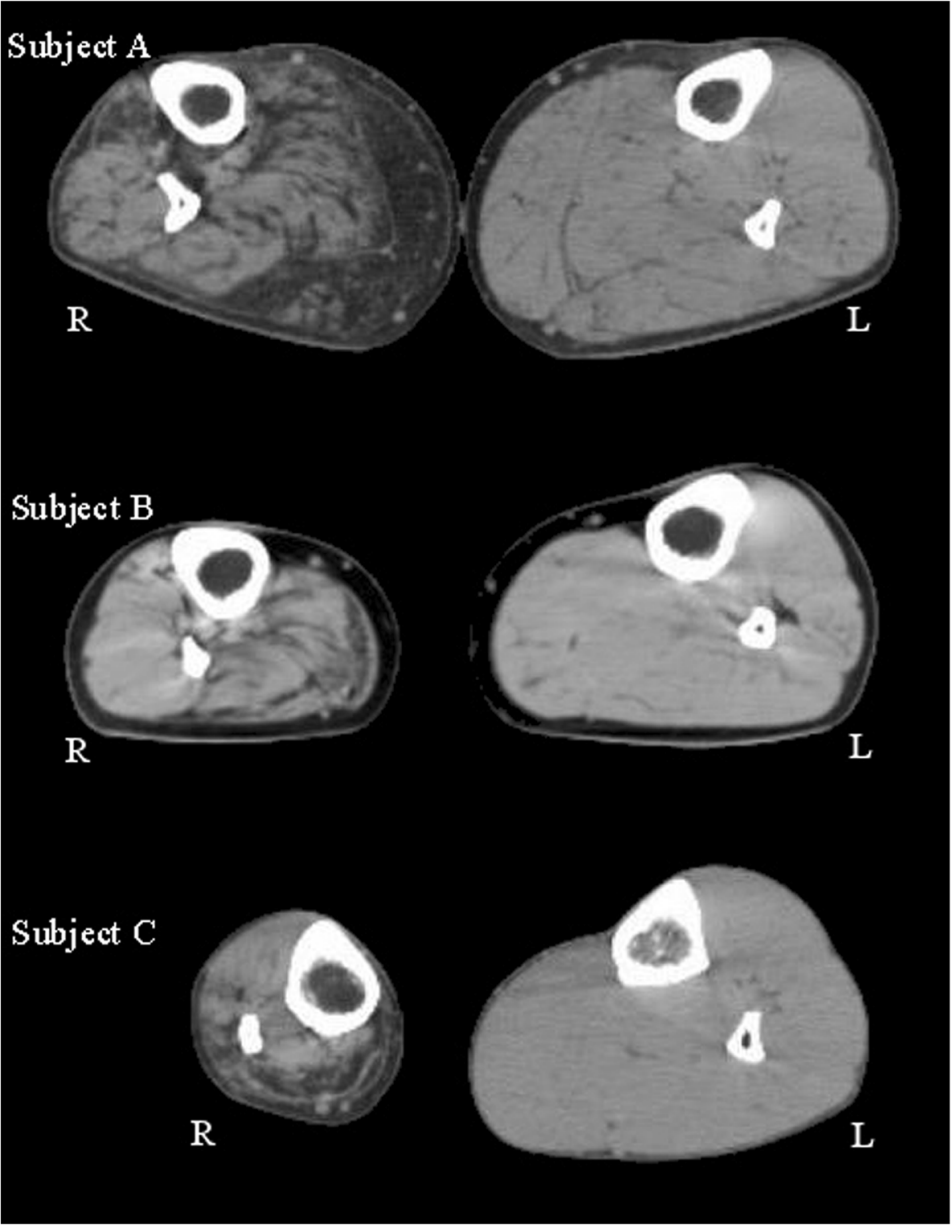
Computed tomography images of the lower right (R) and left (L) leg of the three subjects
